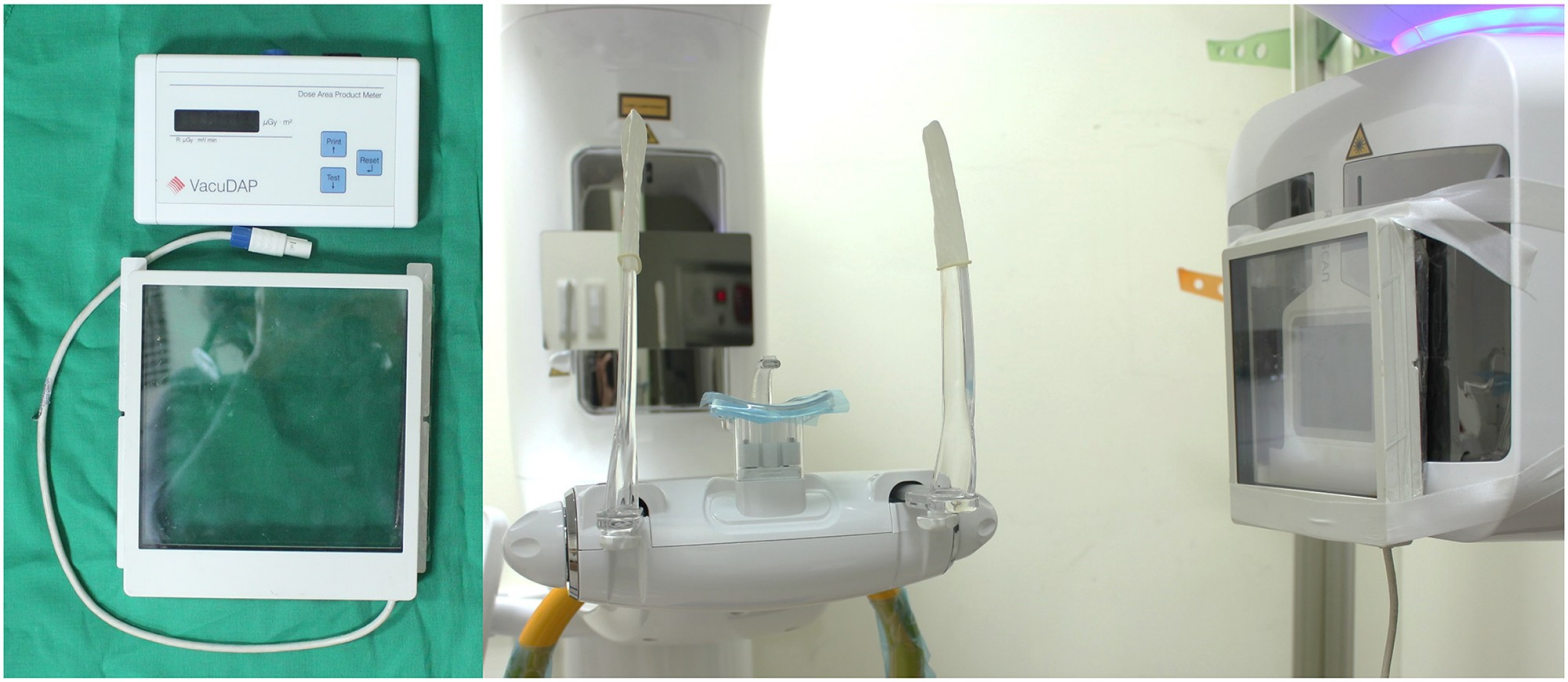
Dose-area-product (DAP) meter (VacuDAP™; VacuTec Meßtechnik GmbH, Dresden, Germany) for input dose measurement. An ion chamber was attached on the surface of the X-ray tube head for the measurements.
